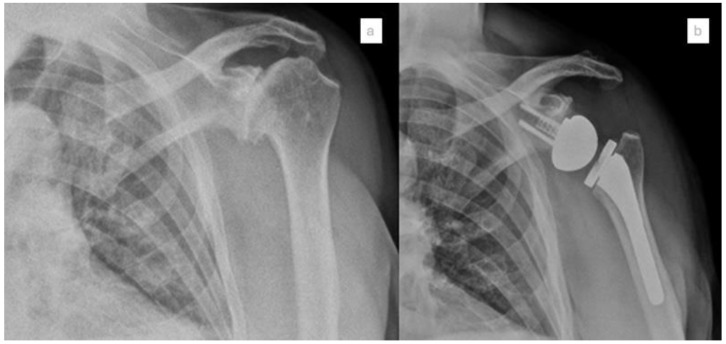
Pre-operative (a) and post-operative (b) X-ray of shoulder arthroplasty performed with GPS navigation.
